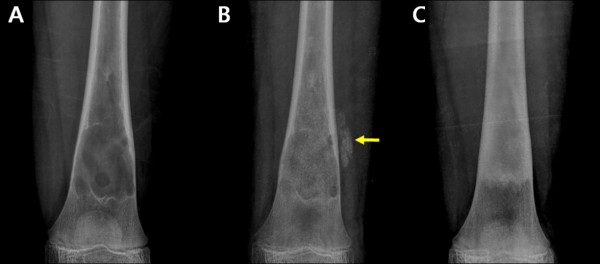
Leakage of DBM. Plain radiographs A) of the distal femur in a 10-year-old girl B) at postoperative one month showing leakage of DBM (arrow) C) at the postoperative 13 months showing complete resorption of extraosseous DBM.
